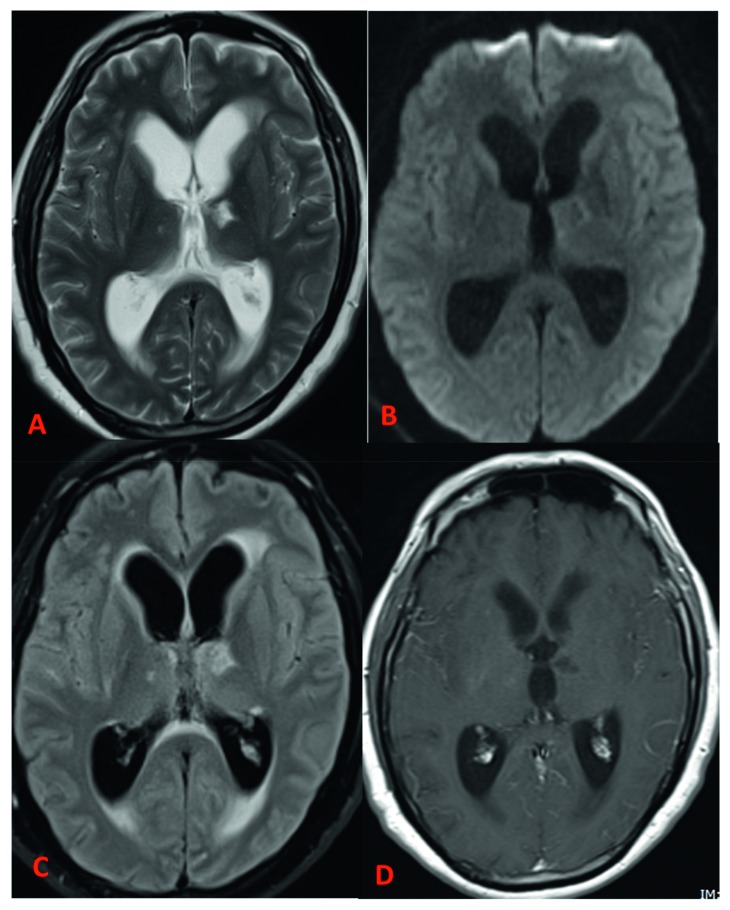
Follow-up MRI brain examination after one month. Axial T2 (A), DWI (B), FLAIR (C), and postcontrast T1-weighted (D) images show significant interval improved leptomeningeal enhancement, resolution of epidural collection (not shown), and interval new communicating hydrocephalus.
